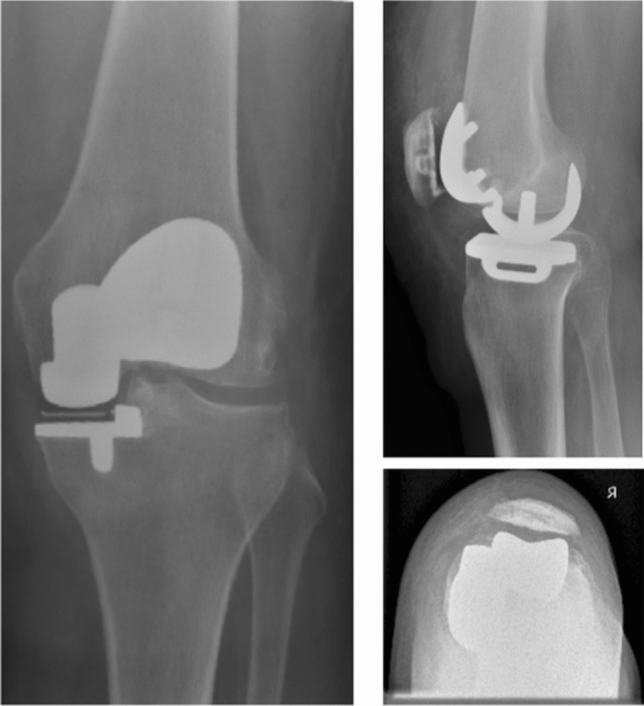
Radiographs depicting a medial bicompartmental arthroplasty: left: anterior–posterior view, top right: lateral view, bottom right: skyline view
